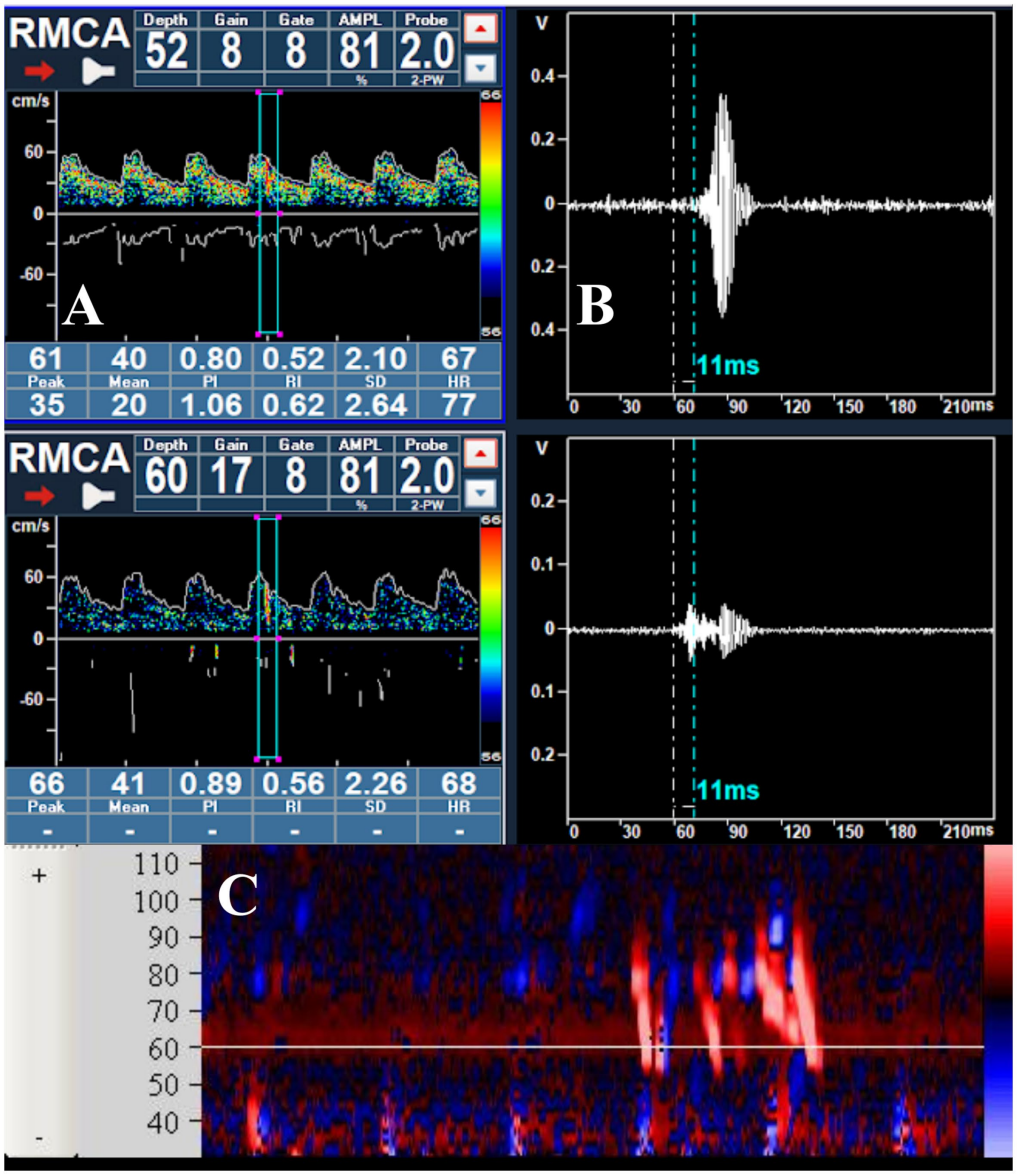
(A) Passage of a microbubble visualized within the Doppler spectrum during the right middle cerebral artery monitoring; (B) raw data of the MB, showing the delay in appearance at a depth of 52 mm; (C) visualization of a series of MBs in the M-mode.
